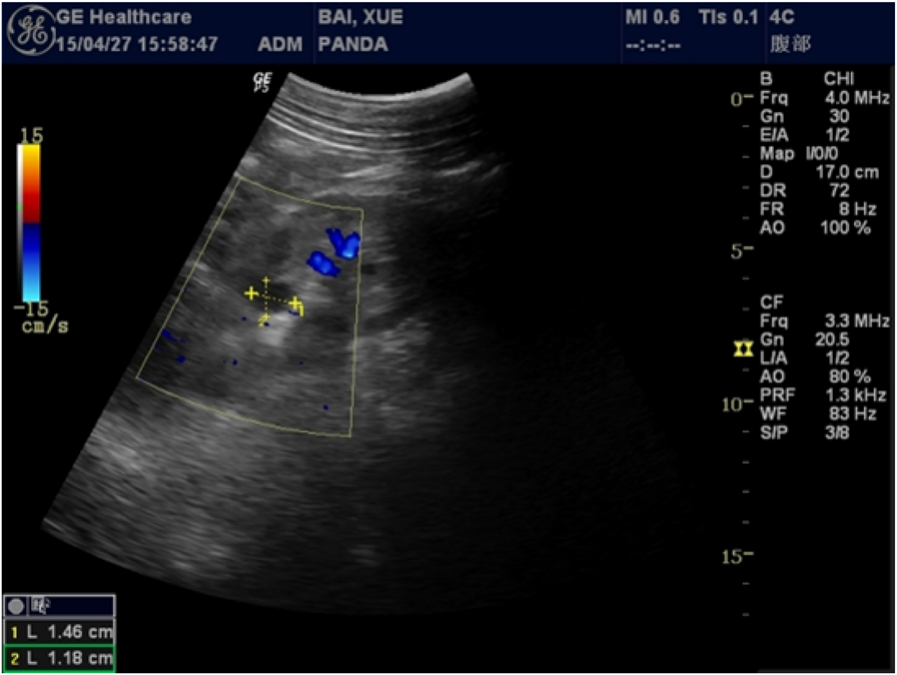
The results of B-scan ultrasound. We found a 1.46 × 1.18 cm cyst in the left kidney. Blue indicates blood flow away from the probe
